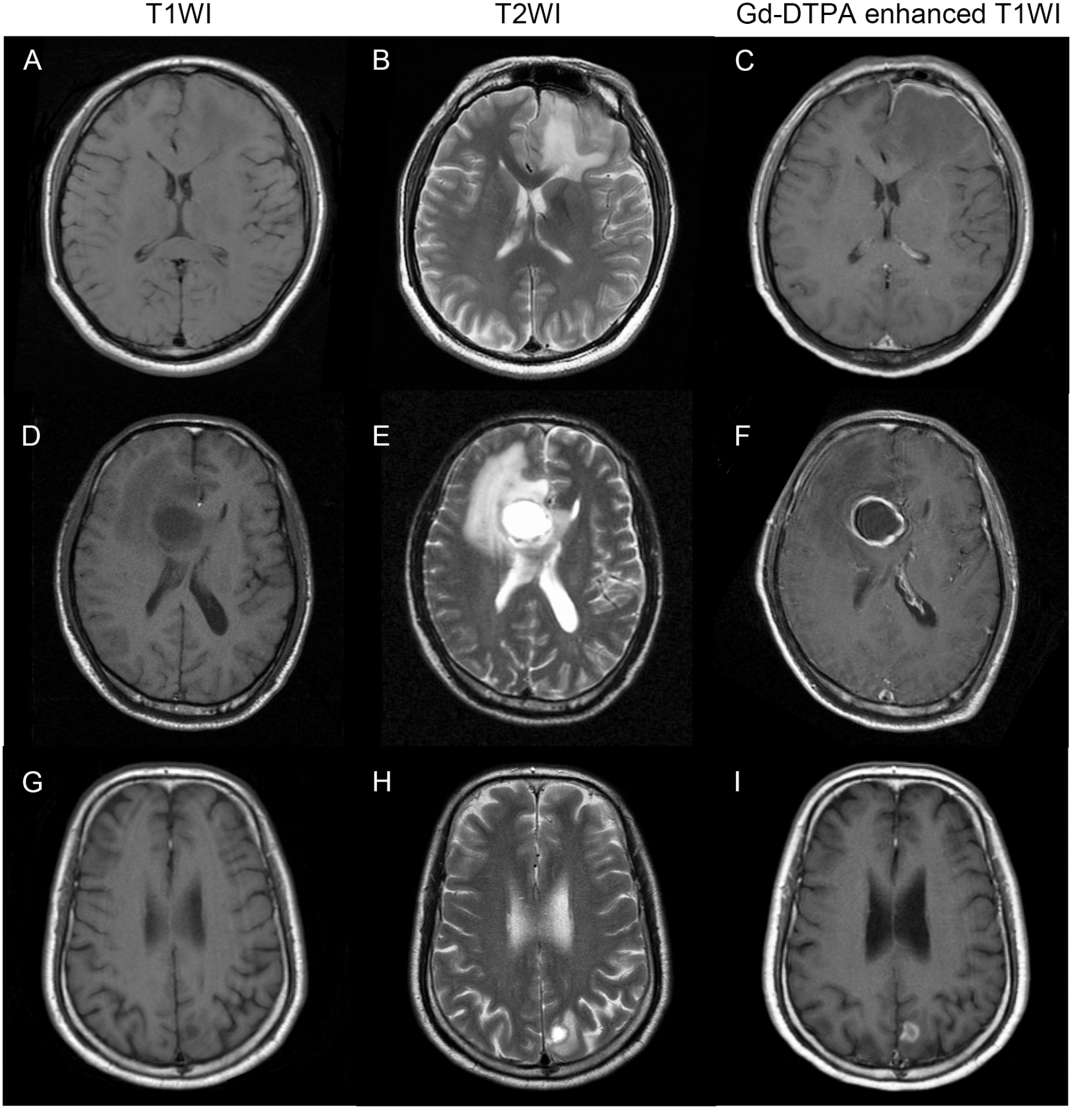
Representative MR images of astrocytoma and solitary cerebral metastasis. A–C: A 39-year-old man with WHO grade I pilocytic astrocytoma without enhancement that is located in the left frontal lobe and in contact with the frontal horn of the left lateral ventricle. D–F: A 47-year-old man with WHO grade III anaplastic astrocytoma that is located in the right frontal lobe, is in contact with the frontal horn of the right lateral ventricle, and exhibits apparent edema and necrosis. G–I: A 50-year-old man with left occipital renal carcinoma metastasis that is not in contact with the lateral ventricle.
